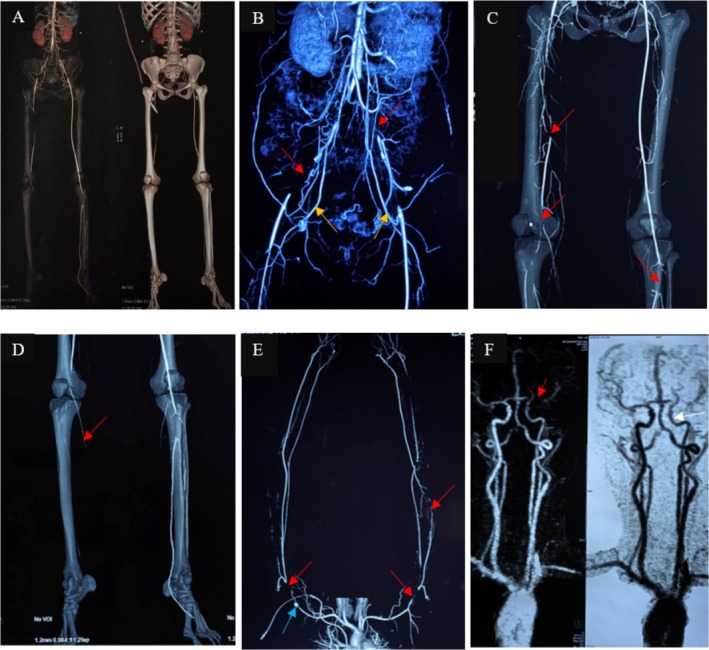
CT angiogram of abdominal aorta and lower limb demonstrated extensive arterial thrombosis, involving proximal left external and internal iliac arteries, mid and distal right common iliac artery with extension into right external and internal iliac arteries (A, B), proximal right mid and lower superficial femoral artery, occlusion of left popliteal artery (C), non‐visualization of distal part of right anterior tibial, posterior tibial and peroneal arteries (D). CT Angiogram of Upper limb arteries demonstrated aneurysm of right mid axillary artery, followed by total occlusion. Besides this, total occlusion of left mid/distal axillary artery, osteo‐proximal brachial artery and bilateral interosseous arteries were also noted (E). MR Angiogram of cerebral vessel showed narrowing of left ICA with non‐visualization of M1 segment of left MCA (F). CT, computed tomography; MCA, middle cerebral artery.
